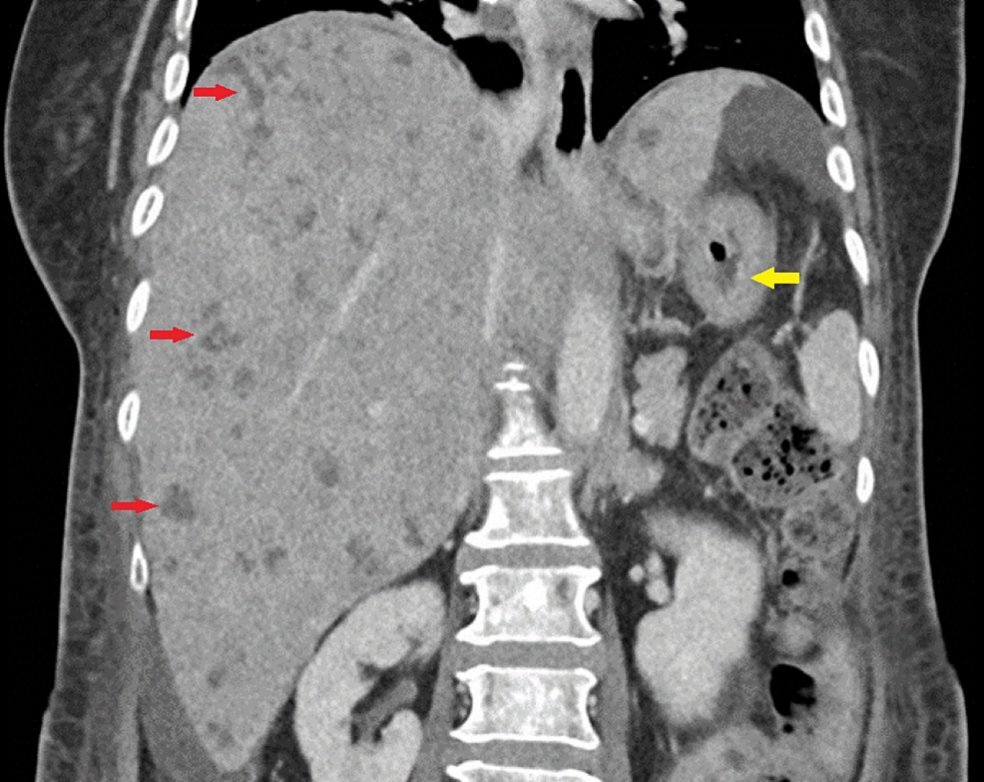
CT of the abdomen showing multiple hypodense hepatic lesions (denoted by red arrows) and mural thickening with mucosal hyperenhancement in the stomach (denoted by yellow arrow)
